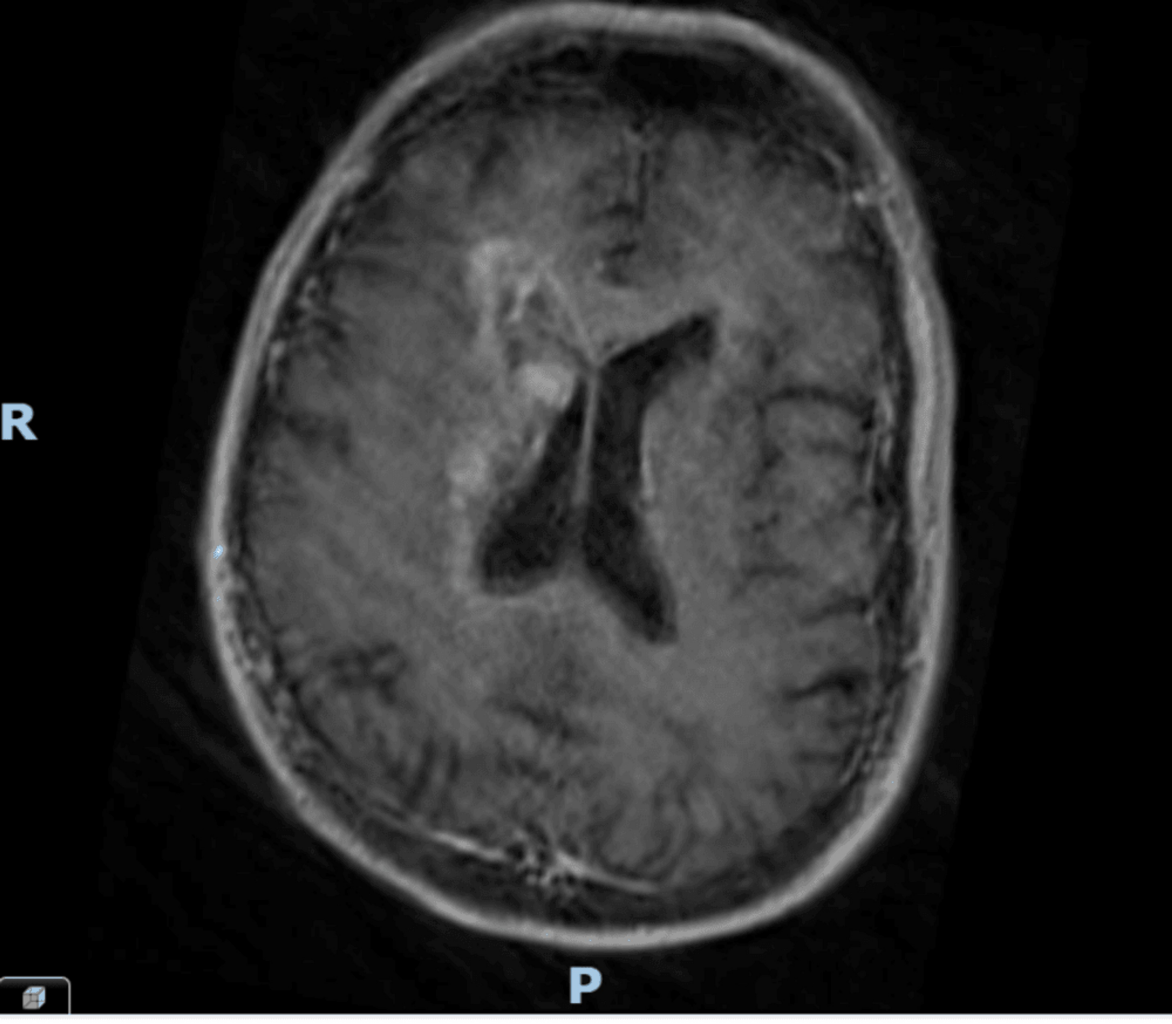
Brain MRI (T1) with contrast Artefactual study due to motion. Abnormal signal and swelling involving the right-sided corpus striatum, internal capsule, thalamus, and periventricular white matter are demonstrated.
